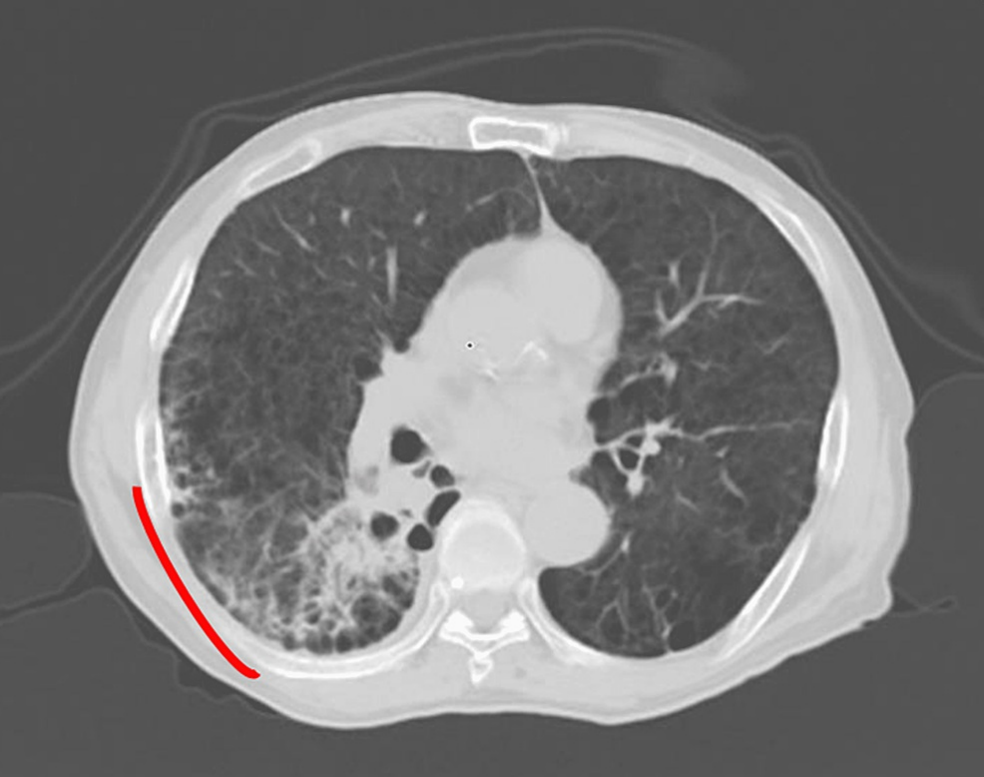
Diagnostic CT image on the same day RP was suggested by the CBCT images RP is shown with a red line. RP - radiation pneumonitis; CBCT - cone-beam computed tomography
